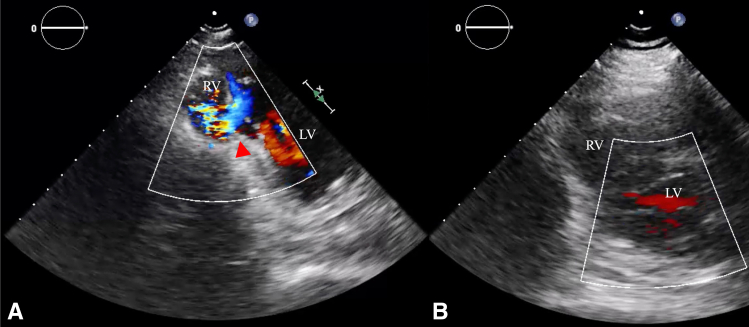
Apical short-axis transthoracic echocardiography: (A) preoperative left-to-right shunt across an apical ventricular septal defect (*arrowhead*); (B) postoperative absence of residual shunt. *RV*, Right ventricle; *LV*, left ventricle.
